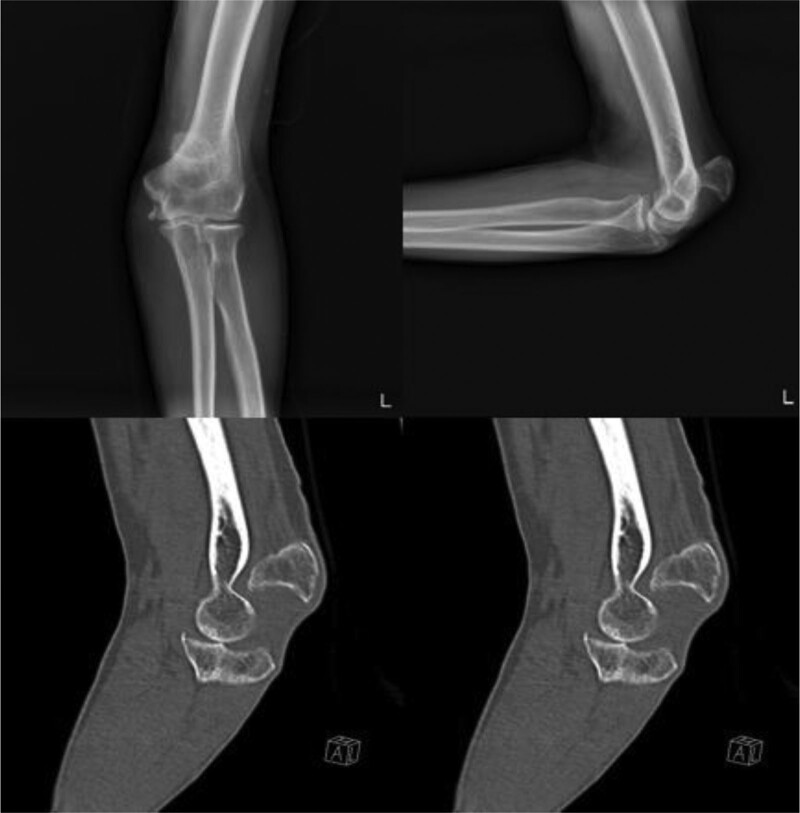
Preoperative X-ray and computed tomography showing olecranon nonunion fracture with separation.
